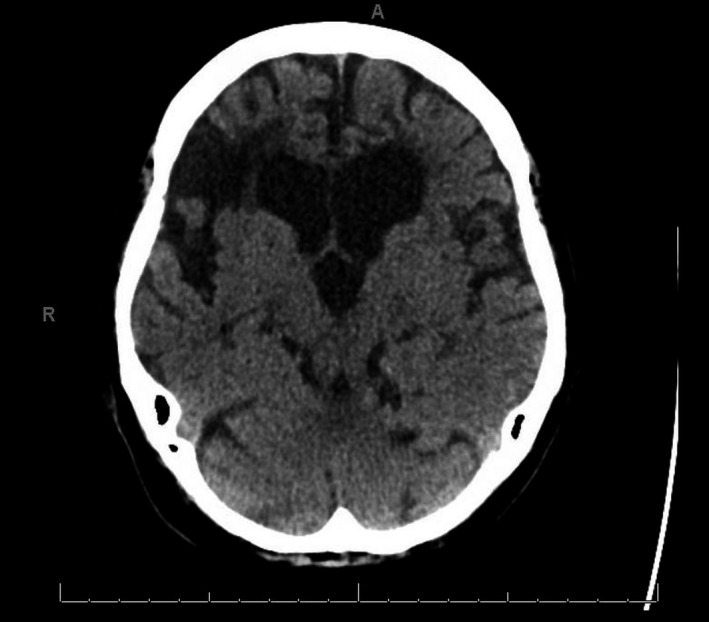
Computed tomography of the head showing bilateral old ischemic infarcts but no acute intracranial pathology.
